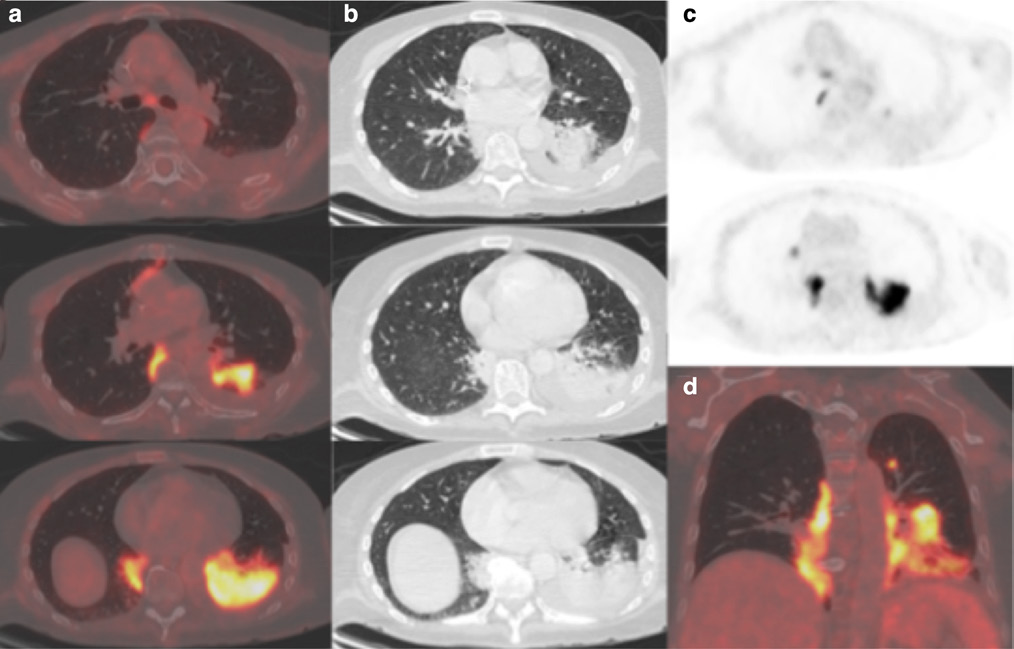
(a) Axial fused thoracic ^18^FDG-PET/CT, (b) axial low-dose CT thorax, (c) ^18^FDG-PET thorax, (d) coronal fused ^18^FDG-PET/CT. The images demonstrate hypermetabolic patchy consolidation in the lower lobes and left upper lobe. Mildly avid subcarinal lymph node. ^18^FDG-PET, 18-fludeoxyglucose-positron emmision tomography.
